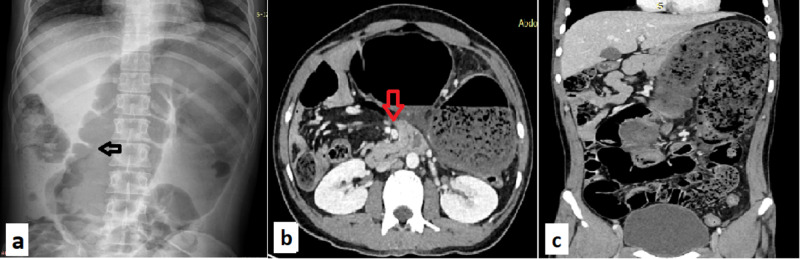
Abdominal radiograph and CECT abdomen. (a) Supine view showing dilated bowel loops in omega conformation with apex in left upper quadrant and haustration (arrow); (b-c) showing ceco-colic volvulus with transportation of mesenteric vessels (arrow). CECT, contrast enhanced computed tomography
